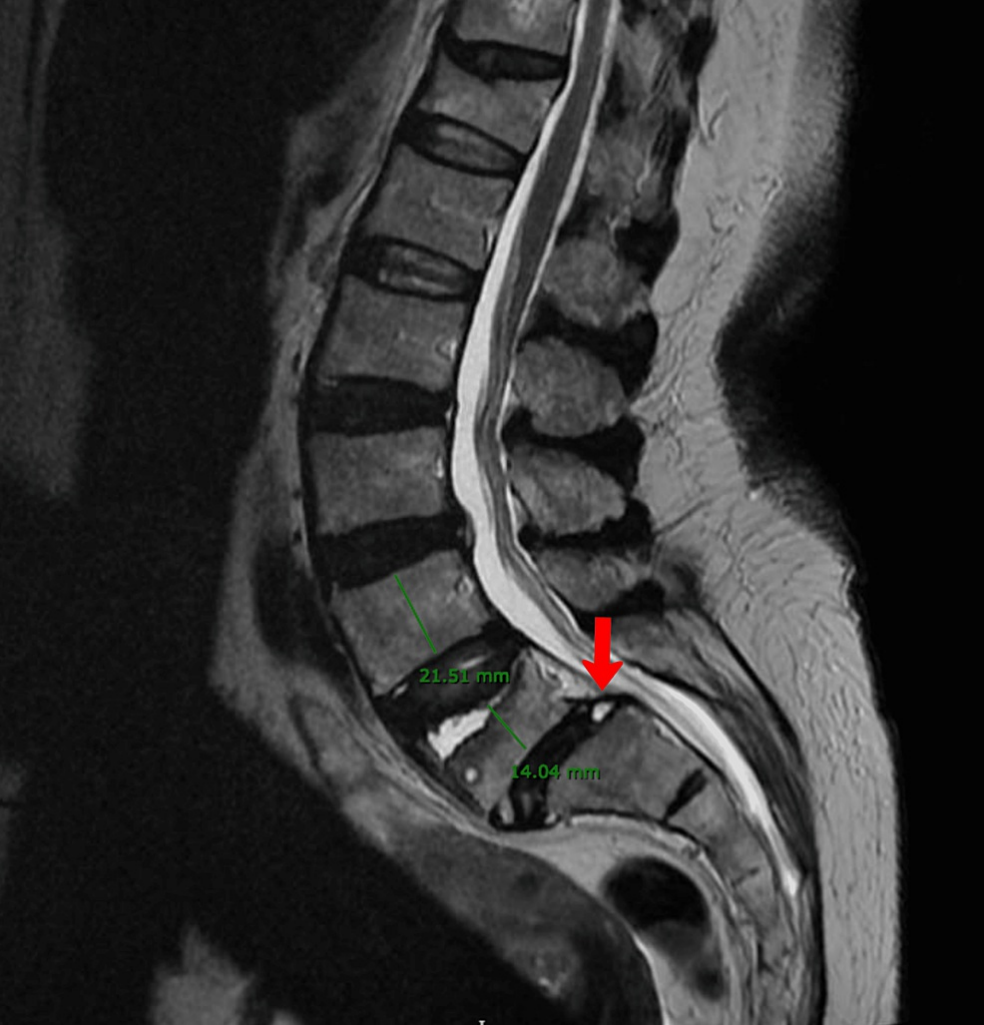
MRI with preserved lordotic curvature with first-degree spondylolisthesis over S1 and decreased height of L5
